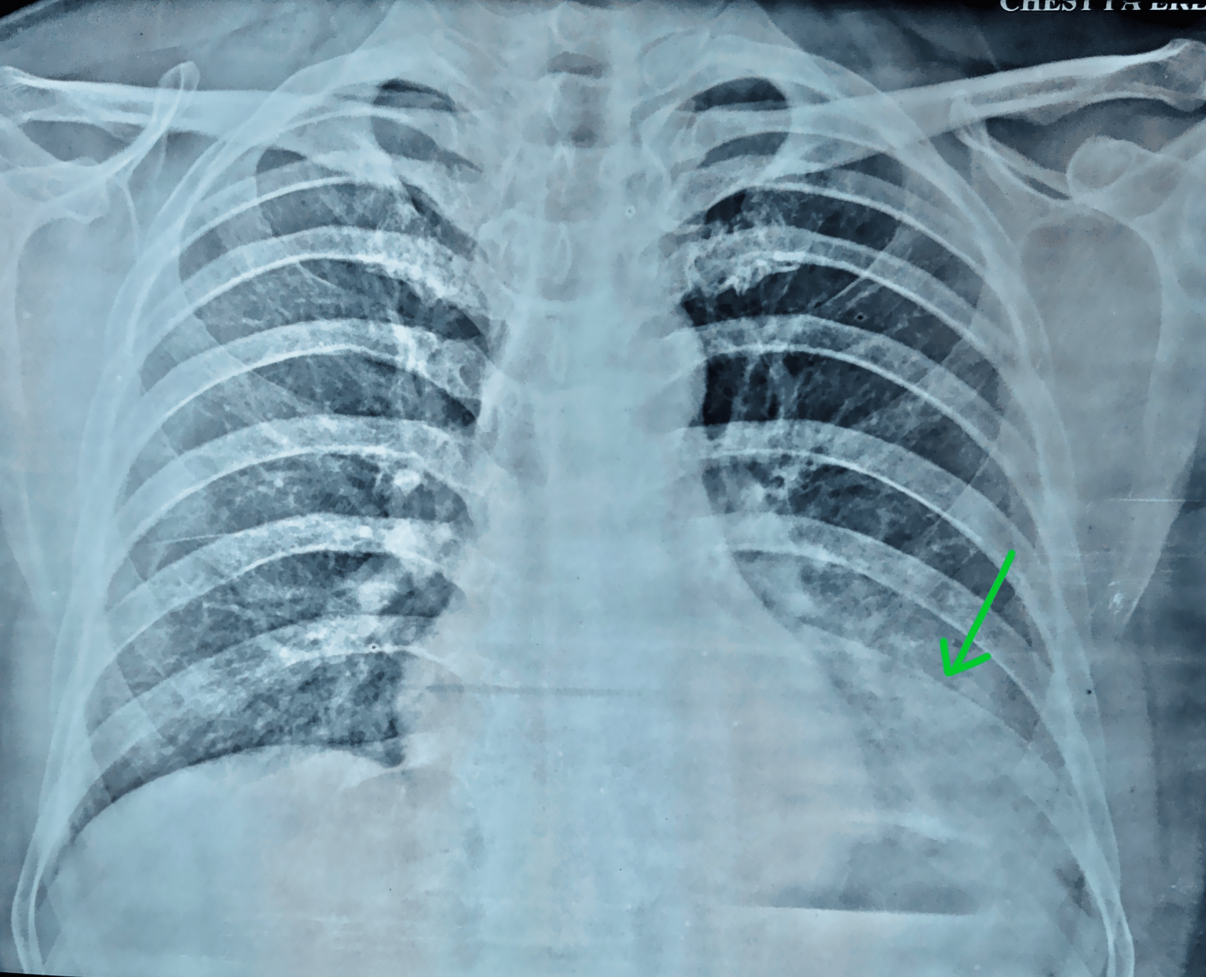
The chest radiograph reveals a left basal pulmonary consolidation (green arrow)
